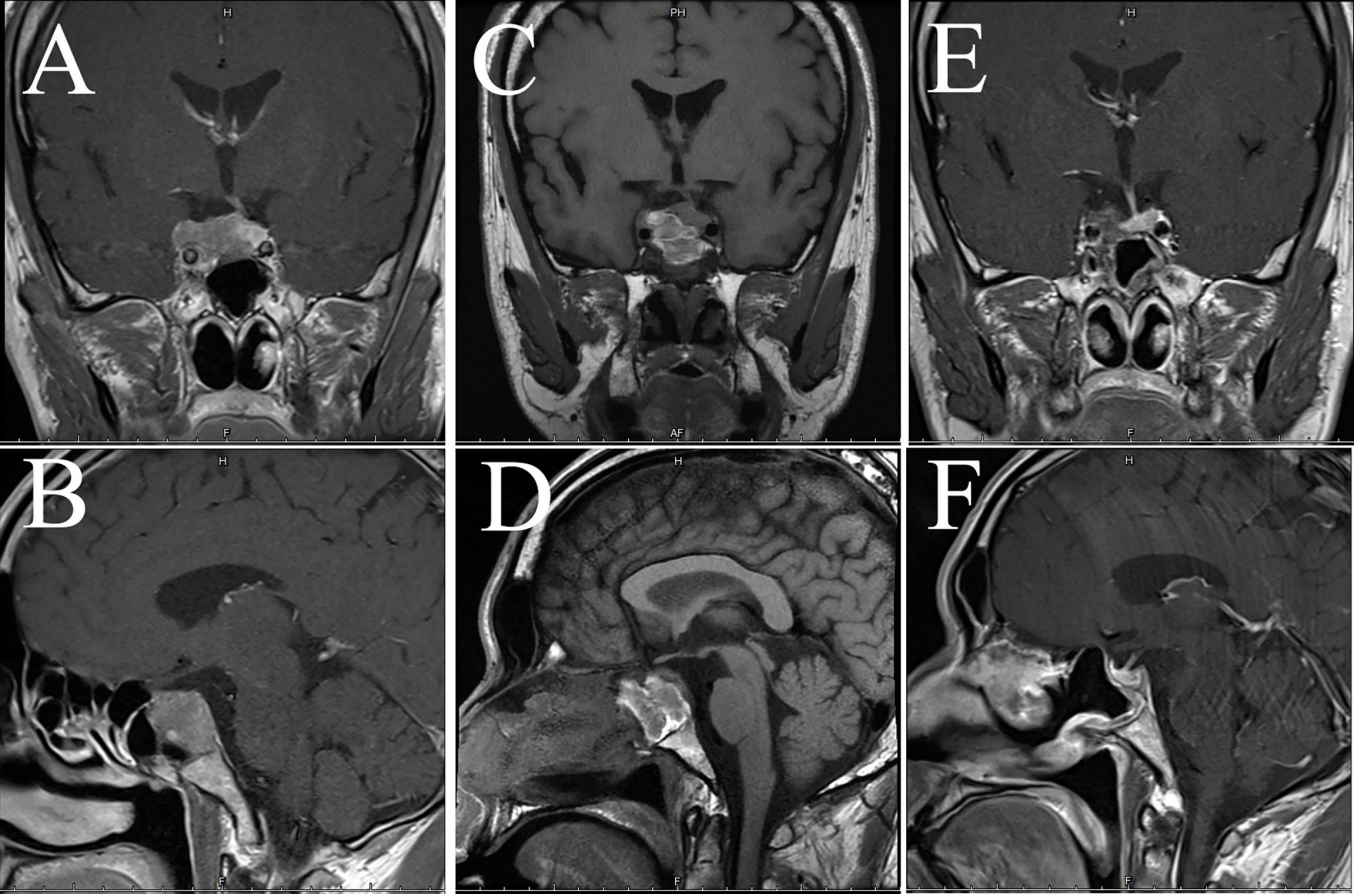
Imaging examination of case 4. The images show coronal and sagittal MRI of the patient’s head preoperatively (A, B), one week postoperatively (C, D), and three months postoperatively (E, F), respectively.
